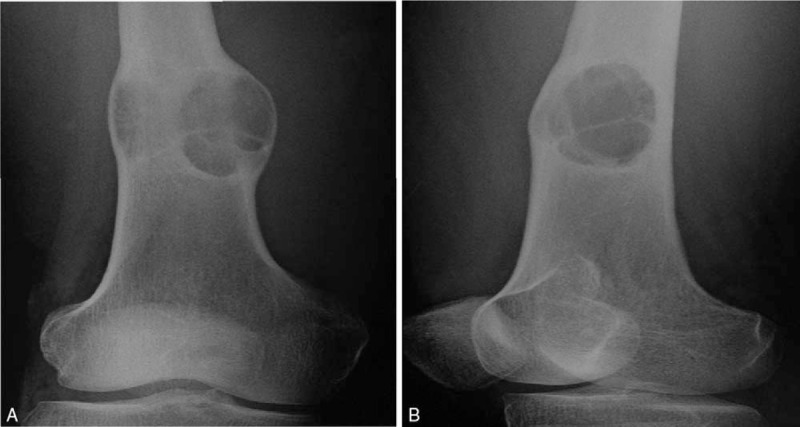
Radiography of the right femur. Anterioposterior (A) and oblique (B) radiograph of the femur revealed an expansive, well-defined radiolucent lesion in the distal segment of the right femur, with sclerotic rim and trabecula inside.
